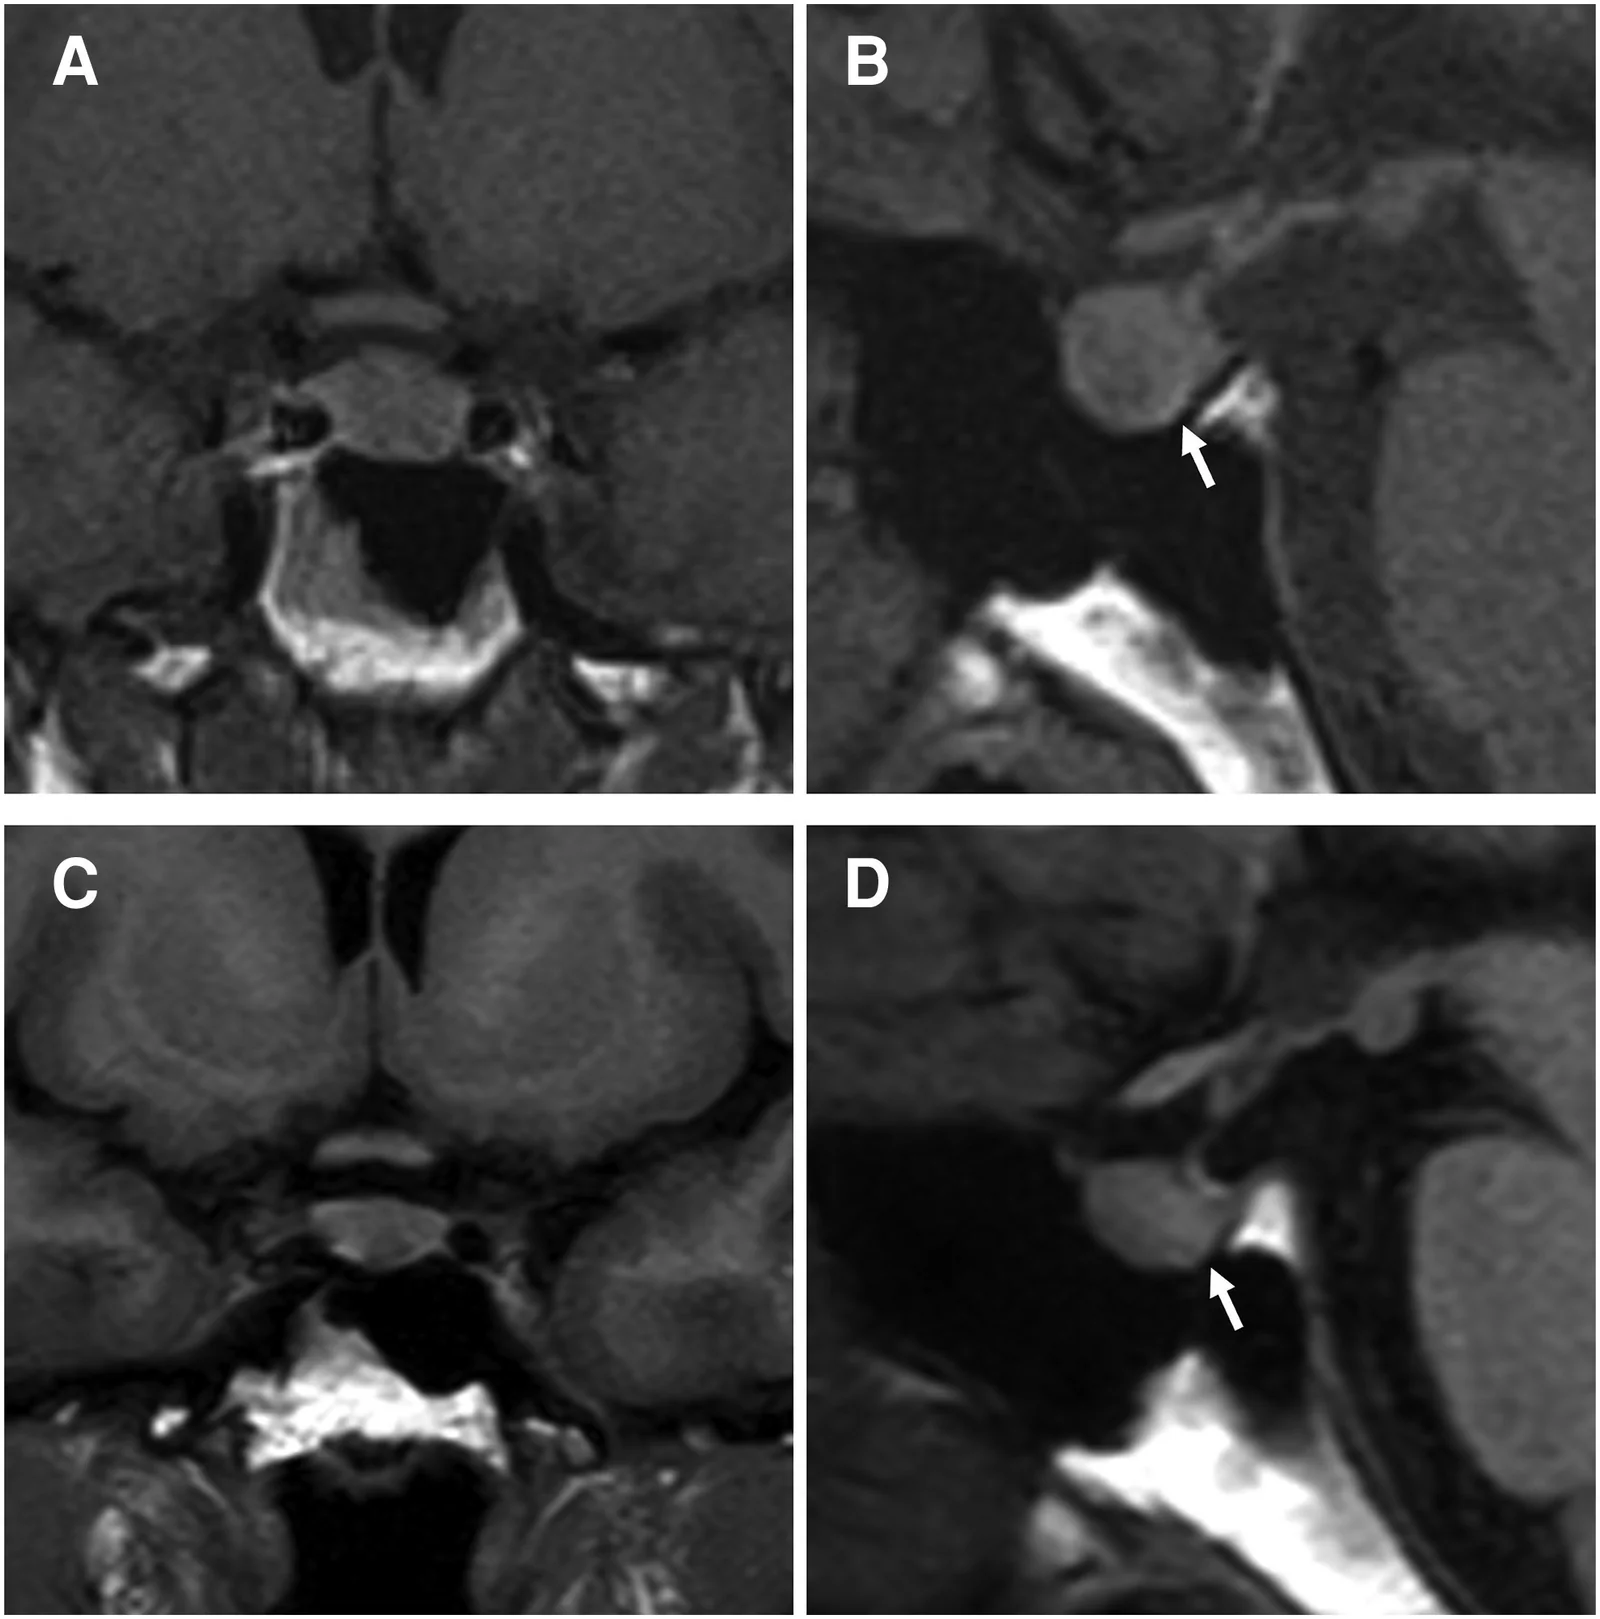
Pituitary magnetic resonance imaging (MRI). (A, B) MRI performed during pregnancy revealing loss of the normal posterior pituitary bright spot (white arrows) with pituitary enlargement (9 mm). (C, D) MRI performed at 6 months postpartum showing improvement in pituitary enlargement (6.5 mm); however, the posterior pituitary bright spot remained absent (white arrows).
